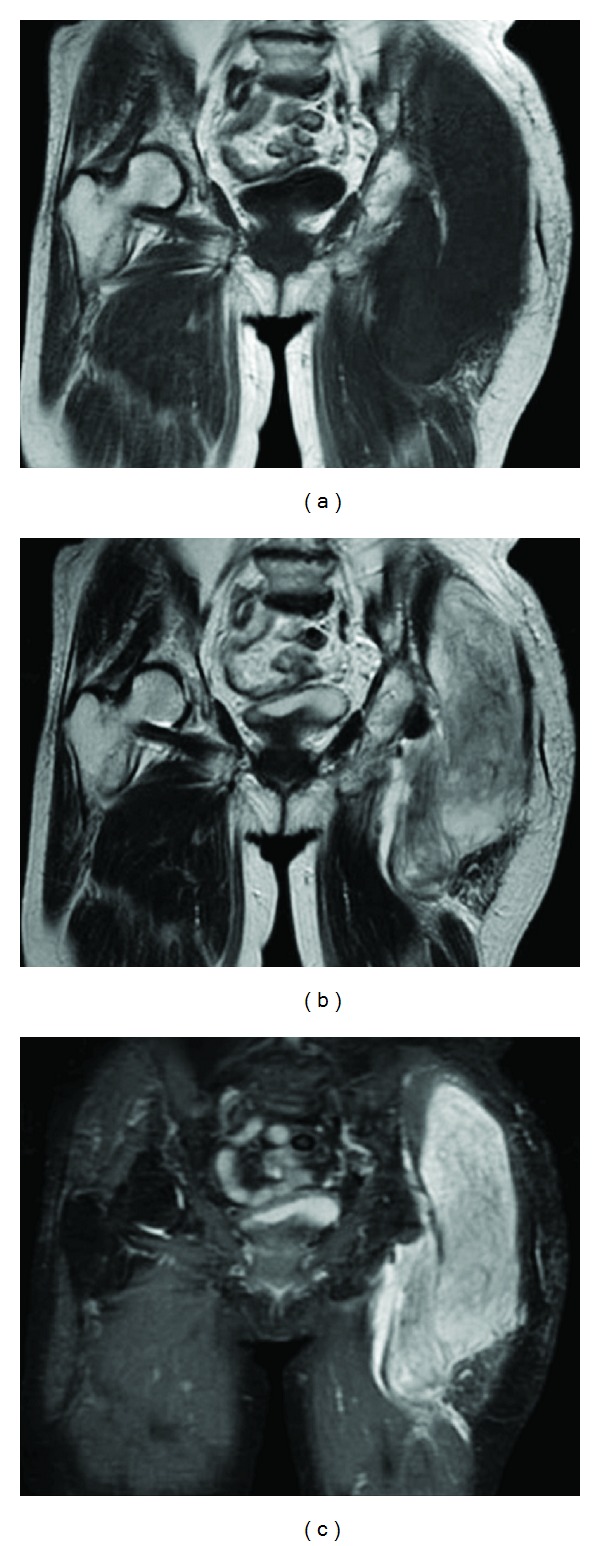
Magnetic resonance images of the left buttock. (a) T1-weighted, (b) T2-weighted, and (c) T2-STIR MR images demonstrated a multilobular intermuscular mass.
